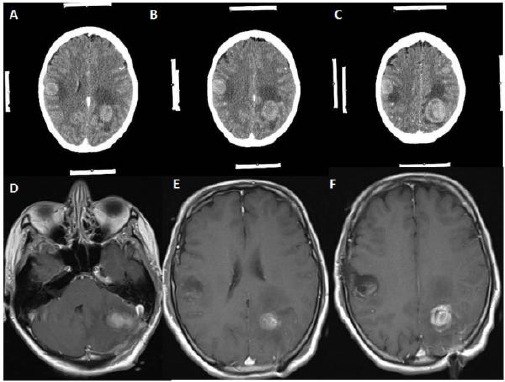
A, B, C (Table 1: Case 5, Metastatic lung tumour): Contrasted axial computerized cranial tomography scans showing that hyperdense oval-shaped lesions with perilesional edematous area at the right frontal lobe and left frontal lobe. Both of these lesions were extracted using stereotaxy technique. D, E, F: Gadolinium-enhanced T1 weighted axial magnetic resonance image scans taken at the postoperative period showing no residual lesion, but there is some postoperative hyperintense changing due to gliosis at the operation regions. I restated that the lesion of the left side of the posterior fossa is not a data for this study, but this lesion also was extracted in gross total manner*.
